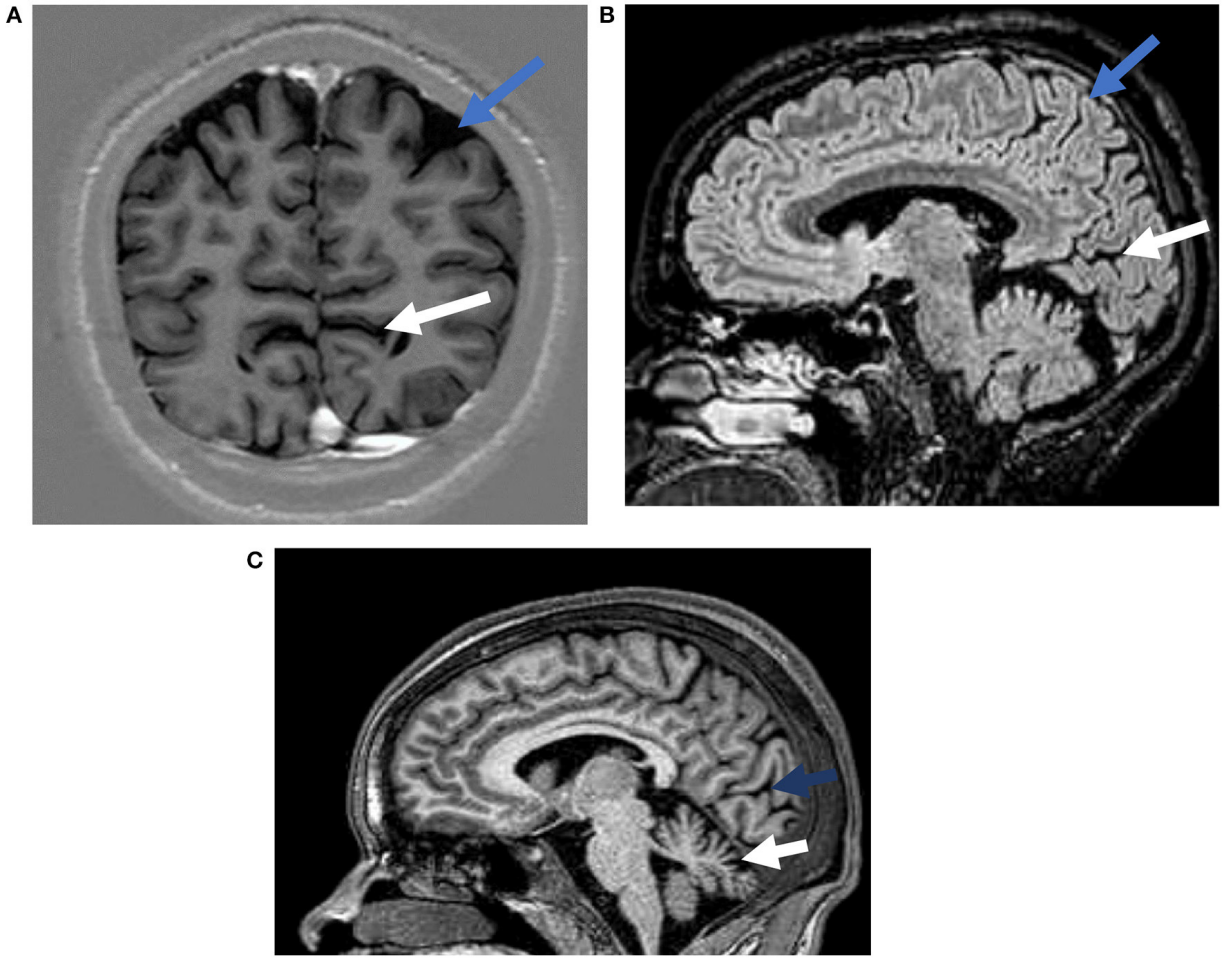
Representative magnetic resonance images from possible OAE epilepsy cases. (A) A T1 weighted inversion recovery image demonstrating occipital atrophy (white arrow) and parietal lobe atrophy (blue arrow). (B) A T2 weighted FLAIR image demonstrating occipital (white arrow), and parietal atrophy (blue arrow). (C) A T1 weighted image showing occipital (blue arrow), and cerebellar (white arrow) atrophy.
